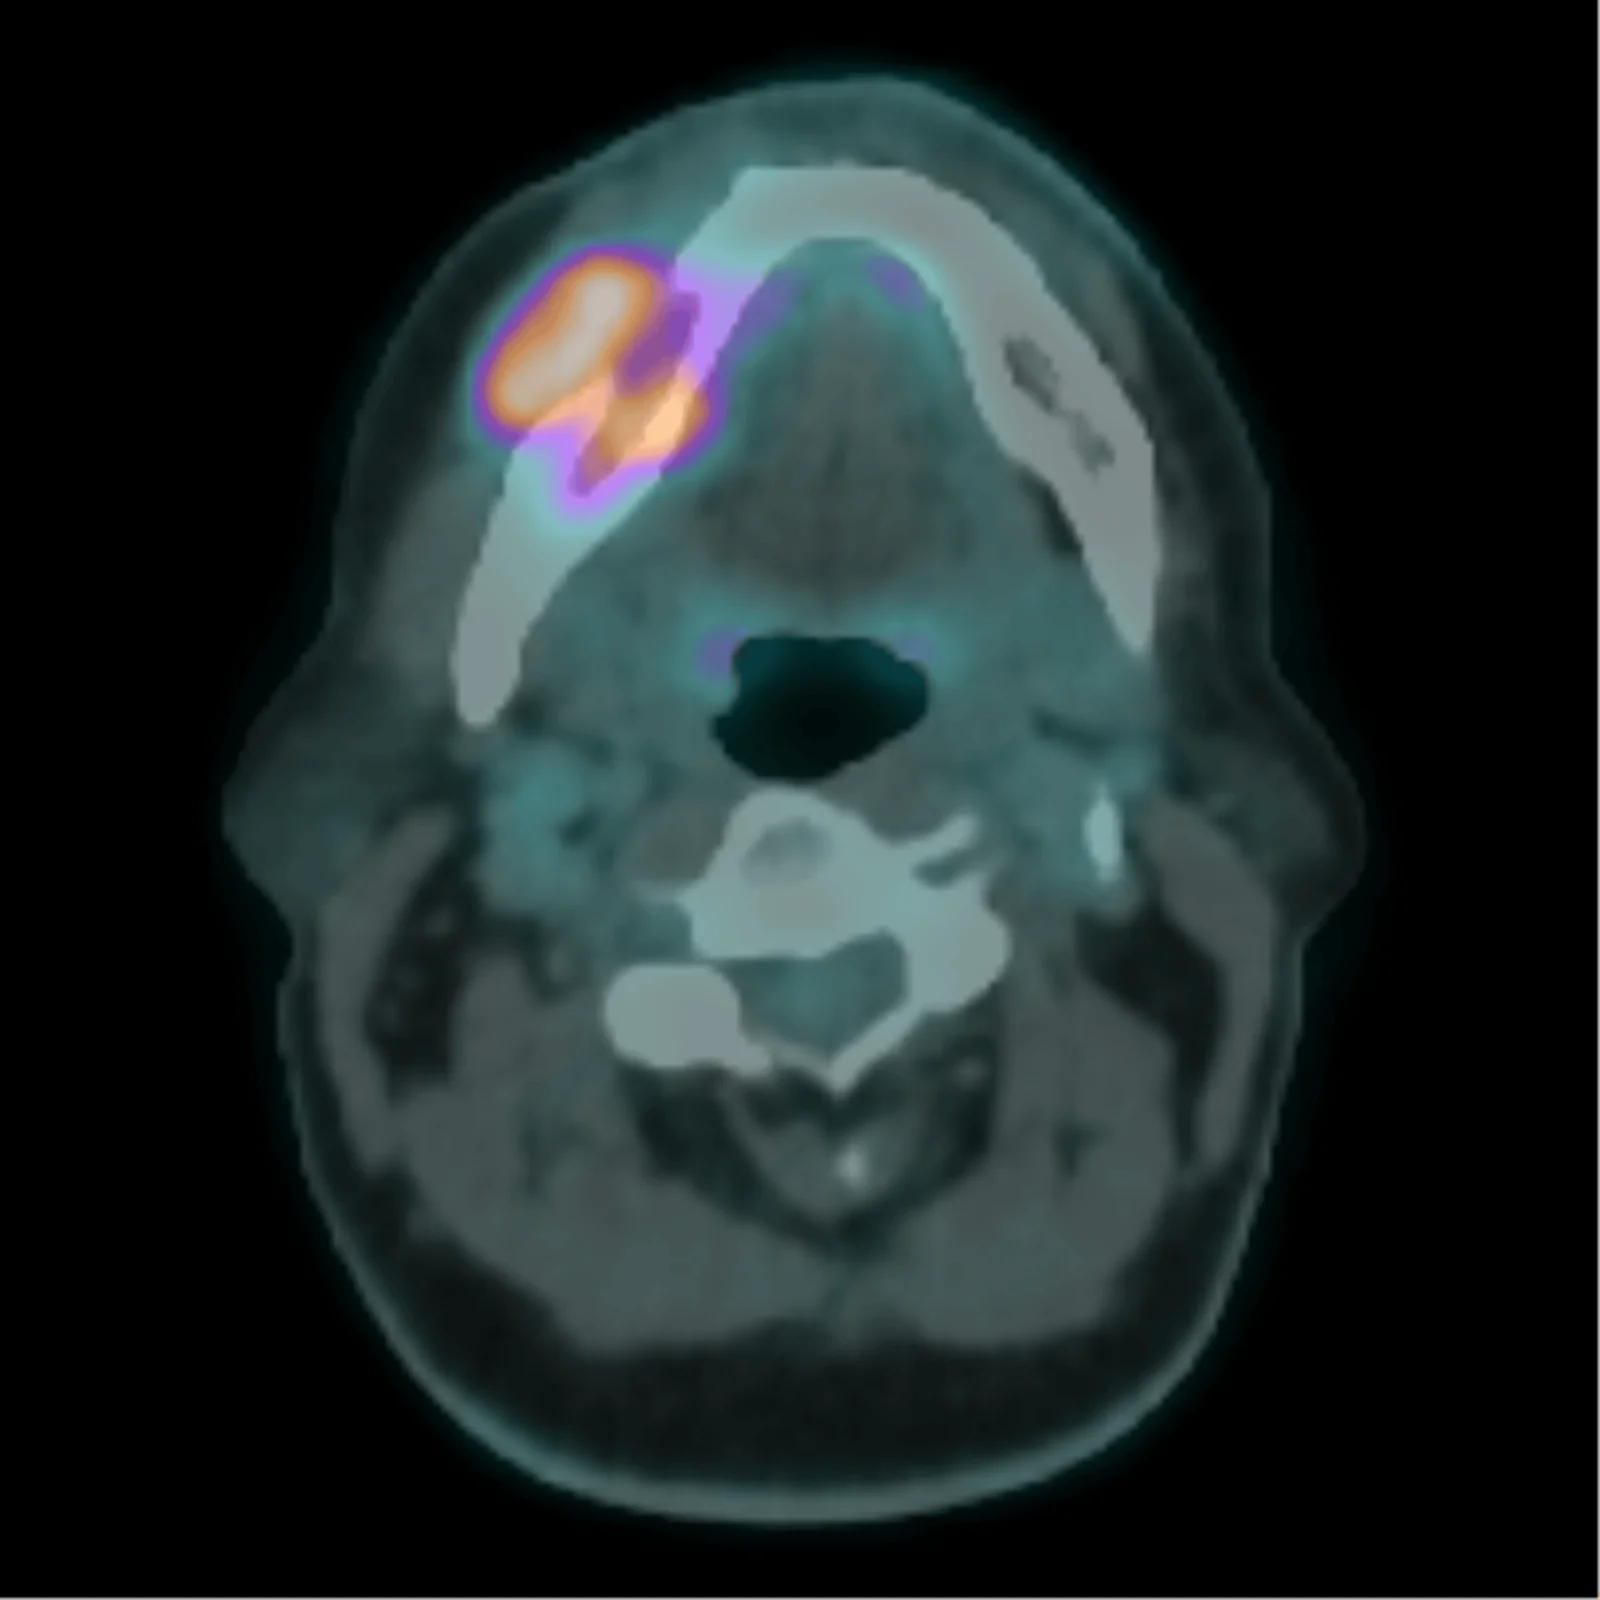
Positron emission tomography/computed tomography image (axial view) depicting the hot spot in the right mandibular molar region
